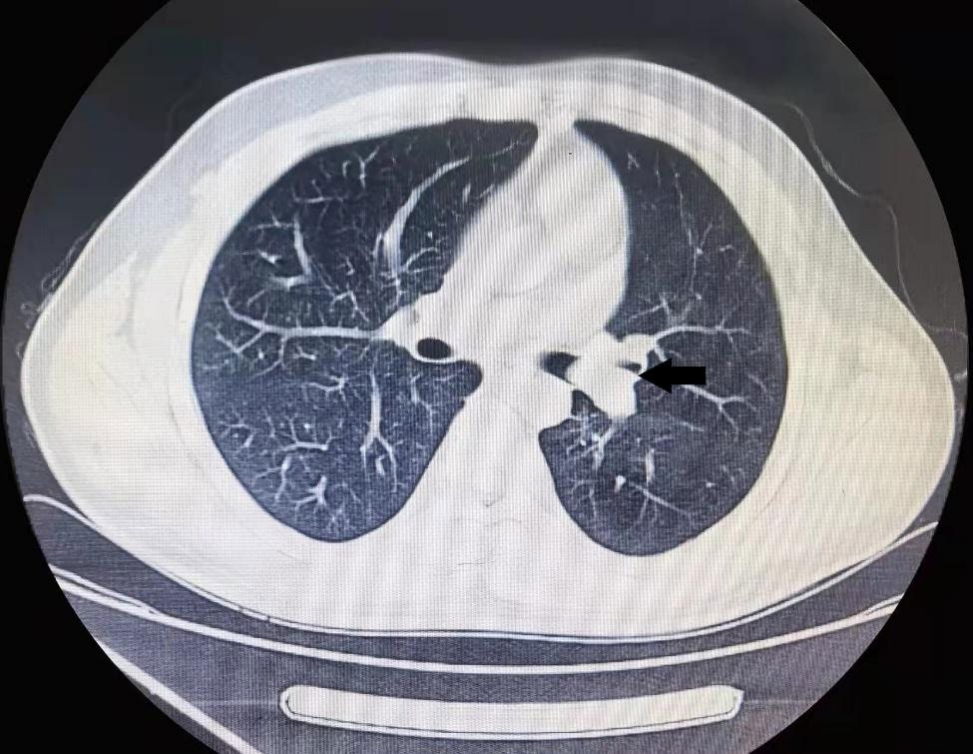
Chest CT revealed that the nodular shaped lesion with abundant blood supply within the LMB. The *arrow* indicates the lesion
